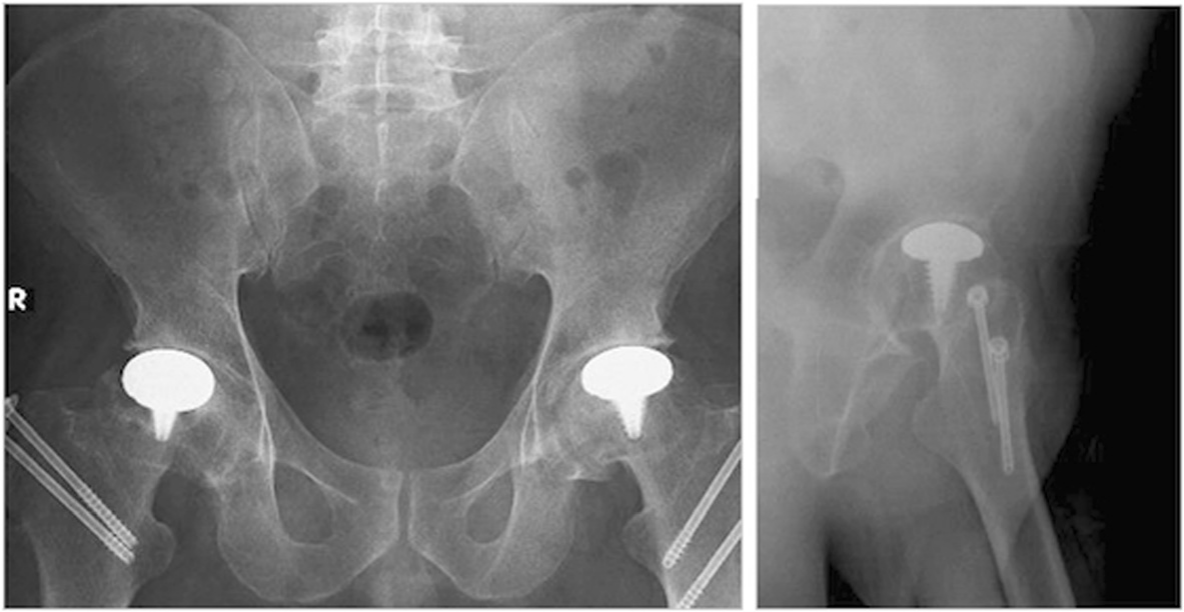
Postoperative X-rays. X-rays of a 37-year-old male patient at postoperative 24^th^ months.
